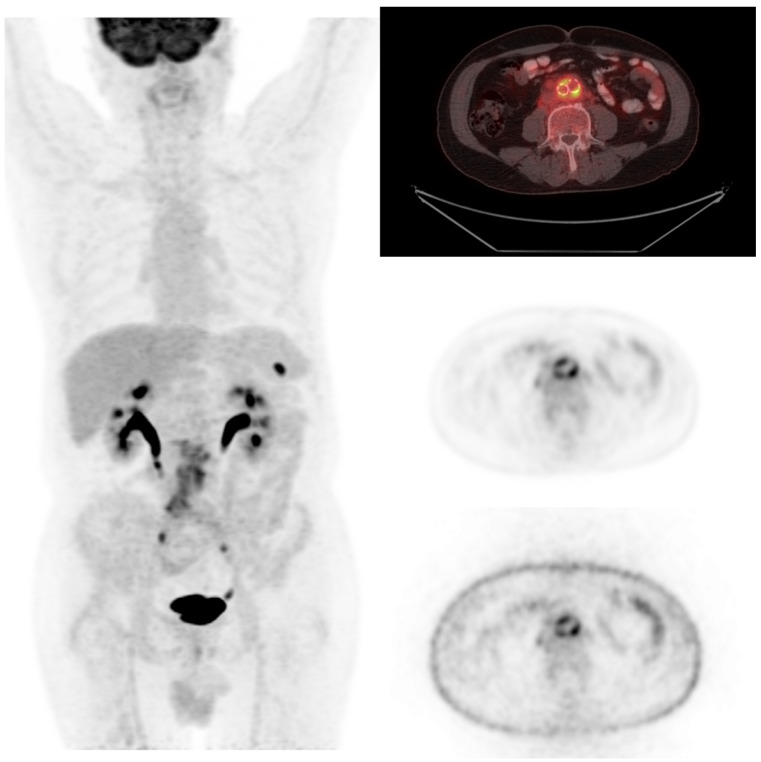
A 69-year-old male patient with a history of abdominal aortic graft placement underwent 18F-FDG PET/CT to evaluate fever of unknown origin. The scan revealed intense FDG uptake around the graft (SUVmax 9.1), indicating an aortic graft infection.
